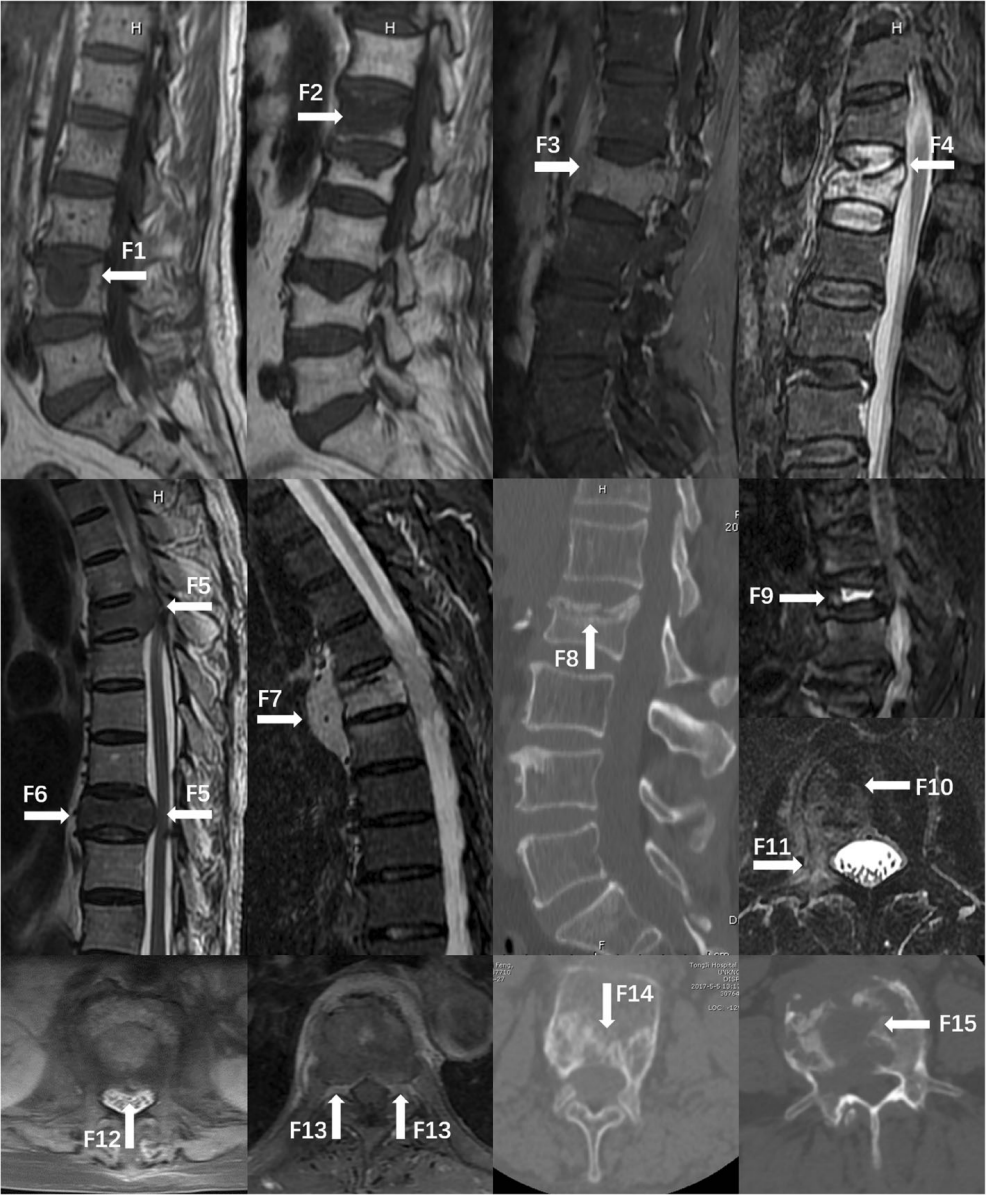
Key radiological magnetic resonance imaging (MRI) and computed tomography (CT) findings. F1 indicates a round vertebral signal intensity change (metastasis of prostate cancer); F2 indicates a band-like vertebral signal intensity change (osteoporotic vertebral fracture [OVF]); F3 indicates a diffuse vertebral signal intensity change (metastasis of lung cancer); F4 indicates a superior sharp protrusion of the posterior wall border (OVF); F5 indicates a smoothly blunt protrusion of the posterior wall border (metastasis of bladder cancer); F6 indicates an anterior vertebral convexity (metastasis of bladder cancer); F7 indicates a paravertebral solid mass (lymphoma); F8 indicates a sclerotic band beneath the end plate (OVF); F9 indicates a cleft fluid sign (OVF); F10 indicates an asymmetry in signal intensity (metastasis of kidney cancer); F11 indicates a pedicle involvement (metastasis of kidney cancer); F12 indicates a single-peaked posterior wall protrusion (OVF); F13 indicates a double-peaked posterior wall protrusion (metastasis of prostate cancer); F14 indicates a vertebral fracture without osteolysis (OVF); F15 indicates an osteolytic destruction (metastasis of lung cancer)
